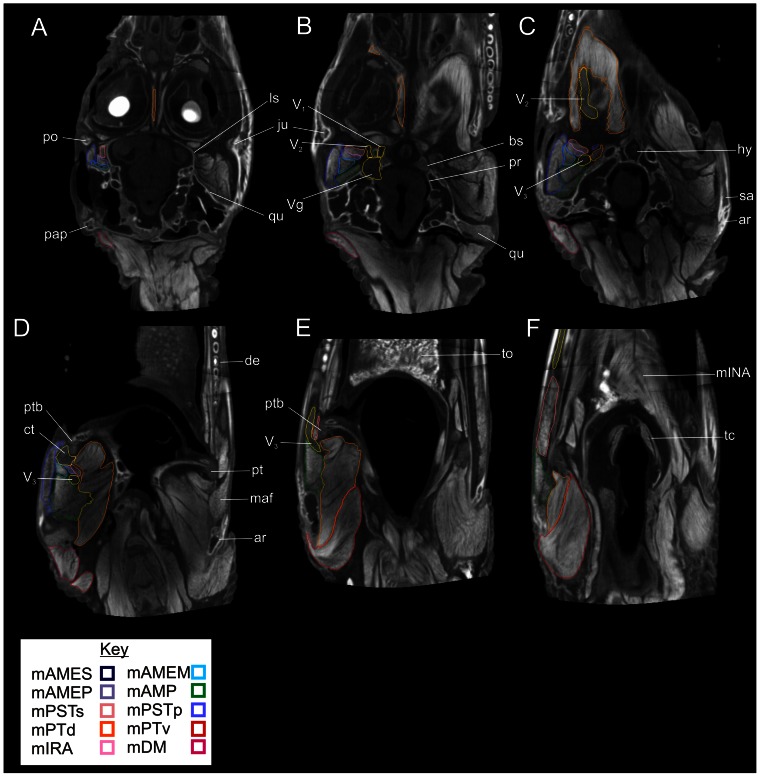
Ventral views of transverse sections through the segmented CT data showing labeled individual right jaw muscles on the left, and unlabeled left structures on the right. A, Slice 312/512; B, Slice 279/512; C, Slice 253/512; D, Slice 210/512; E, Slice 179/512; F, Slice 148/512. Abbreviations: ar, articular; bs, basisphenoid; ct, cartilago transiliens; de, dentary; hy, hypophysis; ju, jugal; ls, laterosphenoid; maf, mandibular fossa; Mf, Meckelian fossa; mINA, m. intermandibularis; pap, paroccipital process; po, postorbital; pr, prootic; pt, pterygoid; ptb, pterygoid buttress; qu, quadrate; sa, surangular; tc, thyroid cartilage; to, tongue; V_1_, ophthalmic nerve; V_2_, maxillary nerve; V_3_, mandibular nerve; Vg, trigeminal ganglion.
